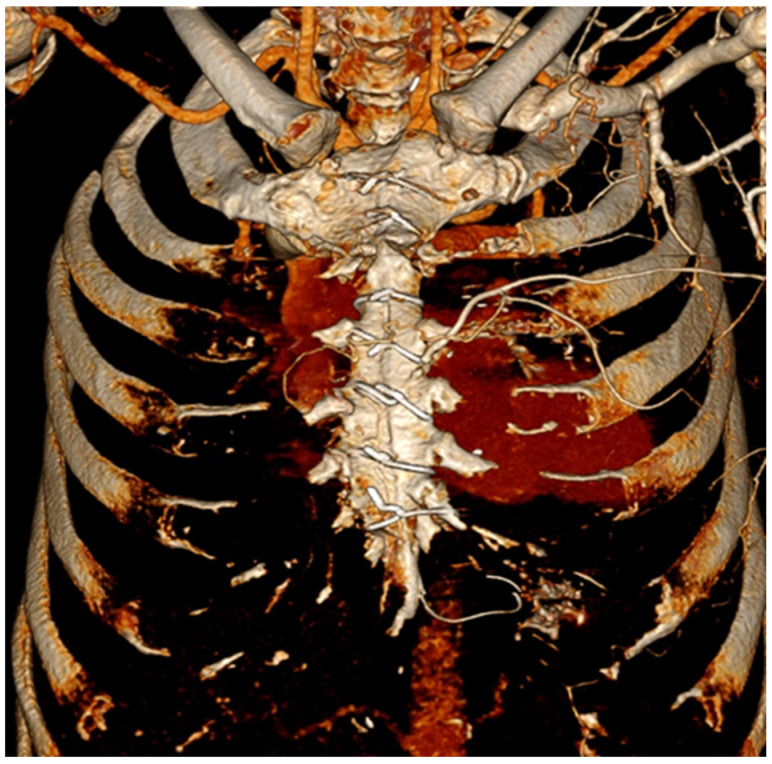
Three-dimensional reconstruction of a CT scan of the sternum after sternotomy. We ask: is it infected?
